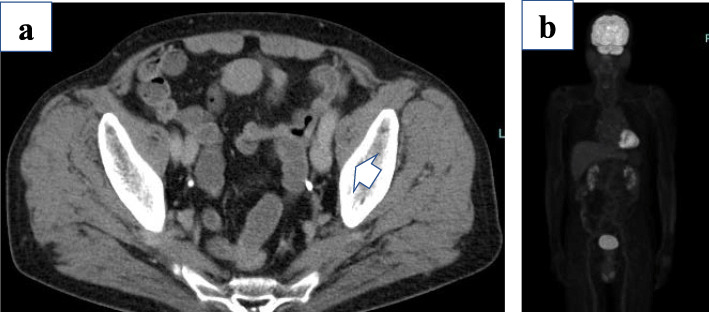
CT and PET-CT findings. a CT revealed an approximately 30-mm enlarged lymph node in the mesentery of the small intestine. b PET showed no FDG uptake
